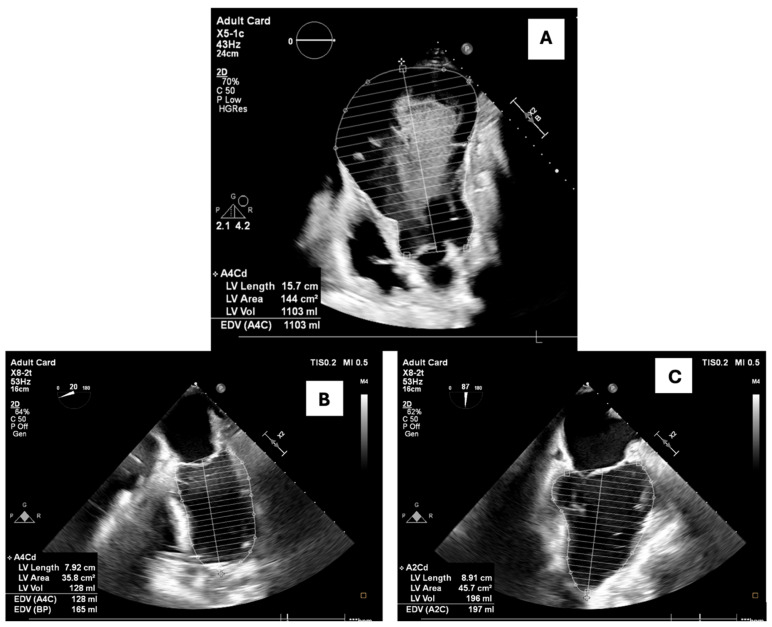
Preoperative and postoperative transthoracic echocardiography. (A) Apical four-chamber view demonstrating a giant post-infarction left ventricular aneurysm associated with massive ventricular dilatation (LVEDV 1103 mL). (B,C) Early postoperative apical four-chamber and two-chamber views showing successful ventricular restoration with recovery of a more physiological left ventricular cavity shape and a substantial reduction in LV end-diastolic volume (LVEDV 165 mL, biplane Simpson method).
